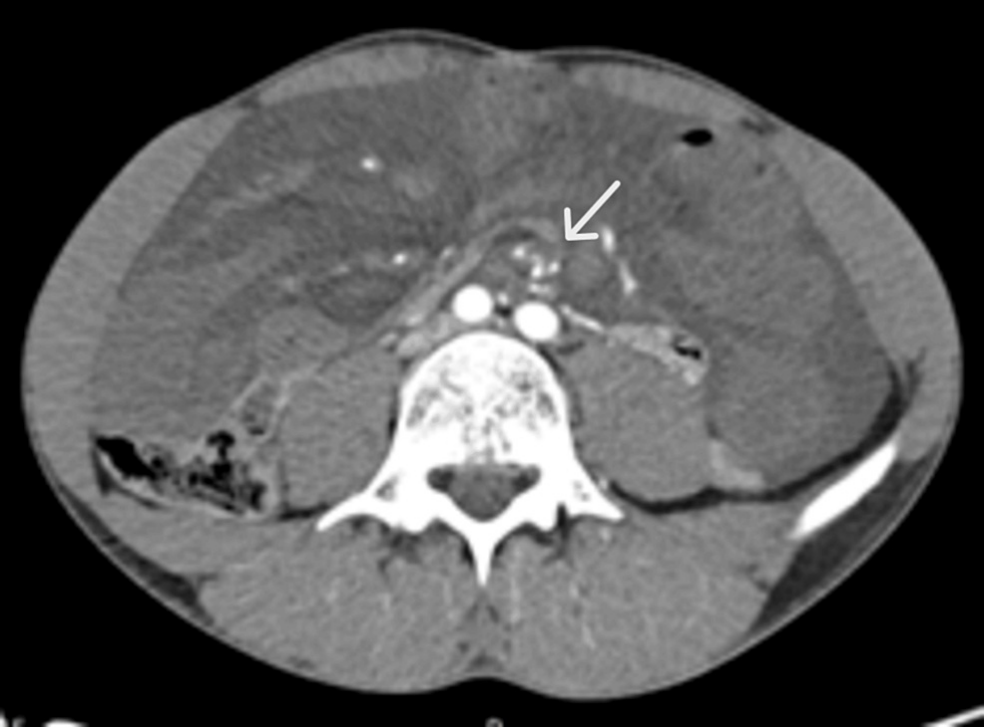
CT Scan axial view: Whirlpool sign (arrow)
